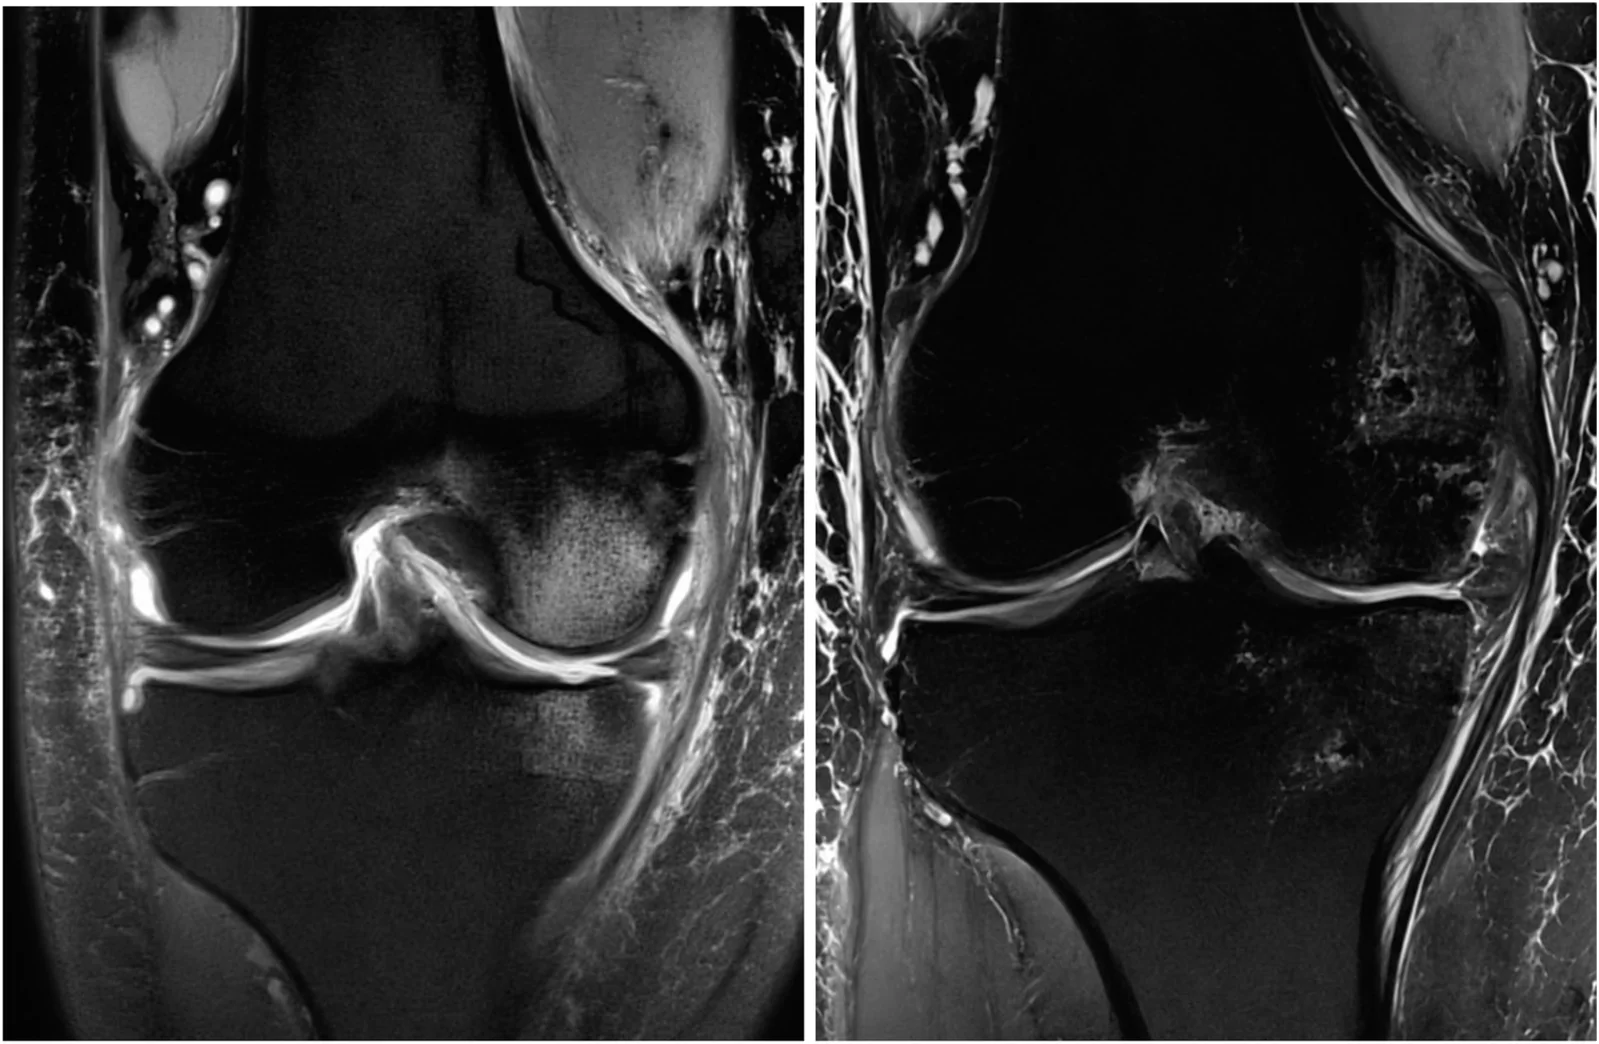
Representative coronal MRI of an enrolled patients undergone subchondroplasty procedure. LEFT) preoperative MRI showing BMLs present at both medial femoral condyle and medial tibial plateau; RIGHT) postoperative imaging, acquired at 24-month follow-up, showing resolution of both the BMLs
